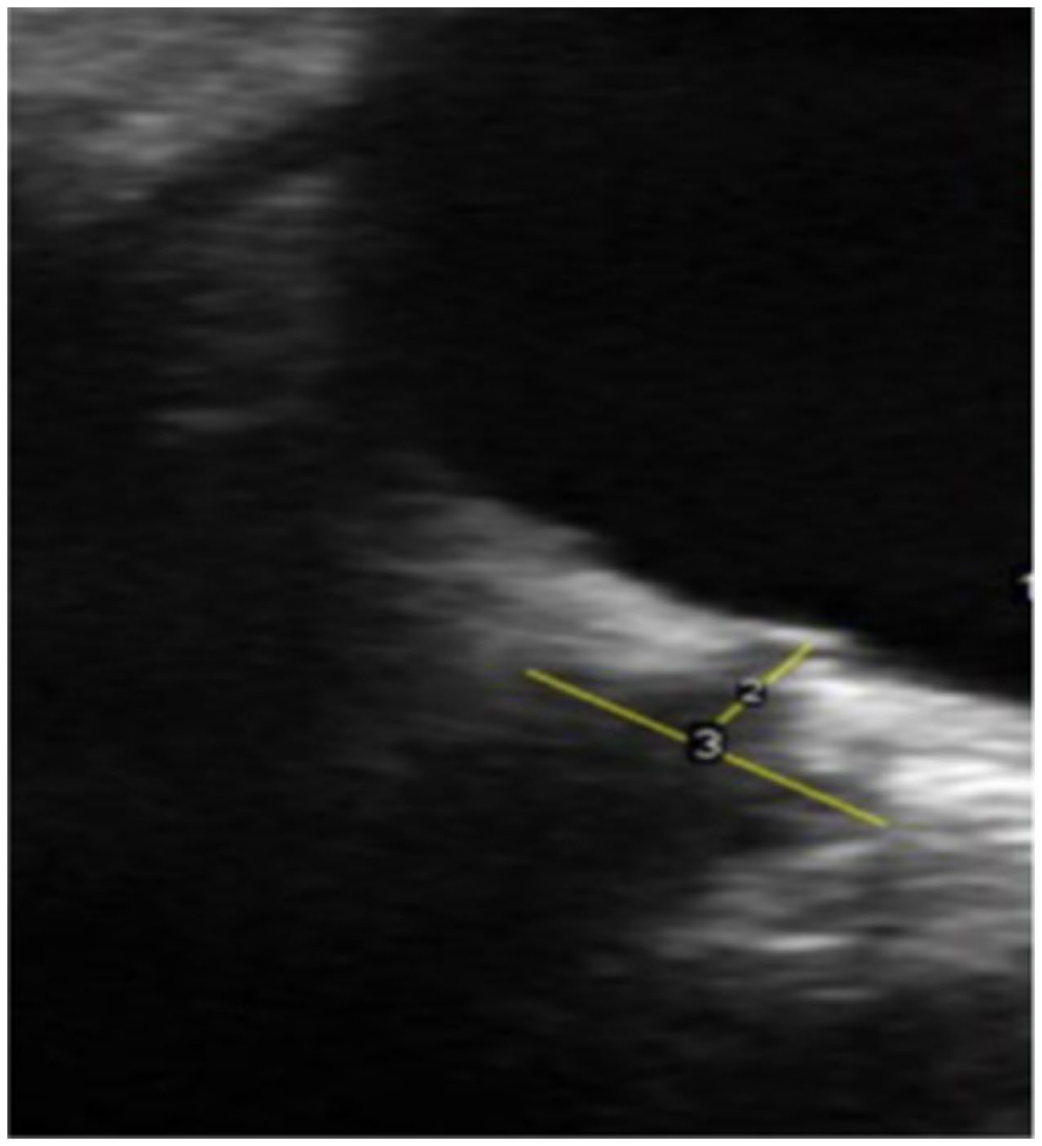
Ultrasound of optic nerve head 3 mm behind the eye socket where optic nerve exits.
